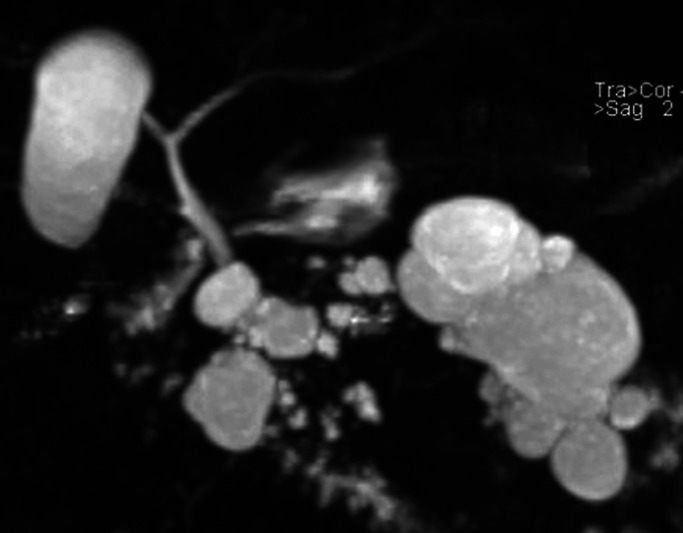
Magnetic resonance imaging with cholangiopancreatography (MRCP) reconstruction of the cystic transformation of the native pancreas.
